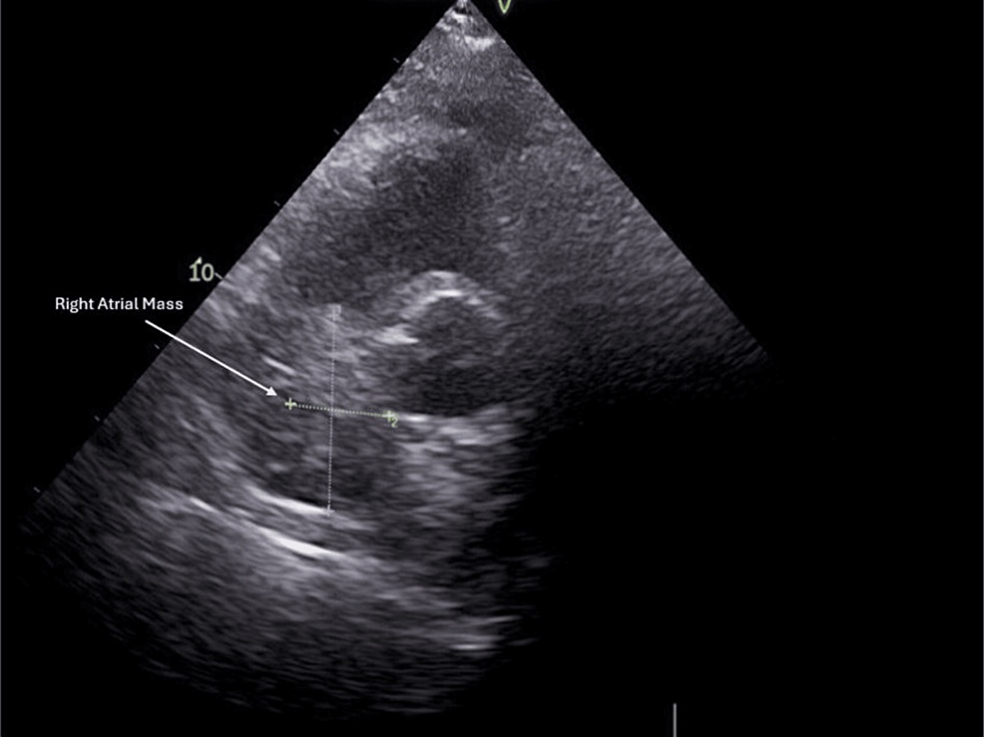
Echocardiography showing a recurrent right atrial mass two weeks after surgical resection of the initial mass
